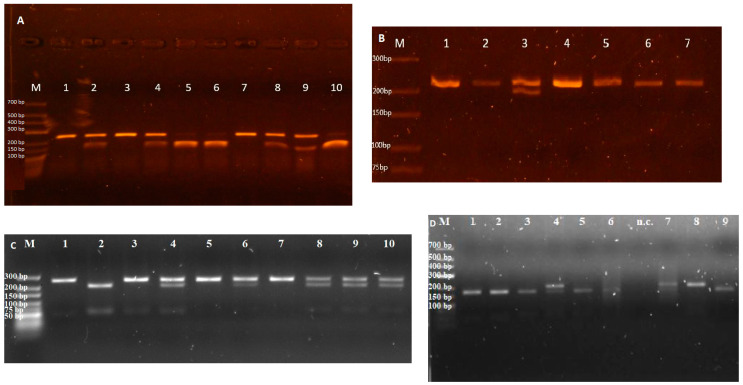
The electrophoresis results of RFLP products. Lane M indicates DNA molecular marker GeneRuler Ultra Low range DNA ladder (A,D) (Thermo Fisher Scientific Baltics, Lithuania); GeneRuler Low range DNA ladder (B,C); (A) PCR-RFLP products for *DMNT1* (rs2228611). Lanes 1, 3, 7—AA genotype; lanes 2, 4, 8–9—AG genotype; lanes 5–6, 10—GG genotype; (B) PCR-RFLP products for *DMNT1* (rs2228612). Lanes 1–2, 4–7—AA genotype; lane 3—AG genotype; (C) RFLP products of *DNMT3A* (rs2276598). Lanes 1, 3, 5, and 7 indicate the CC genotype; lanes 4, 6, and 8–10 demonstrate the CT genotype; lane 2 shows the TT genotype; (D) RFLP products of *DNMT3A* rs752208. Lanes 1–3, 5–6, and 9 indicate the CC genotype; lanes 4 and 7 demonstrate the CT genotype; lane 8 shows the TT genotype.
